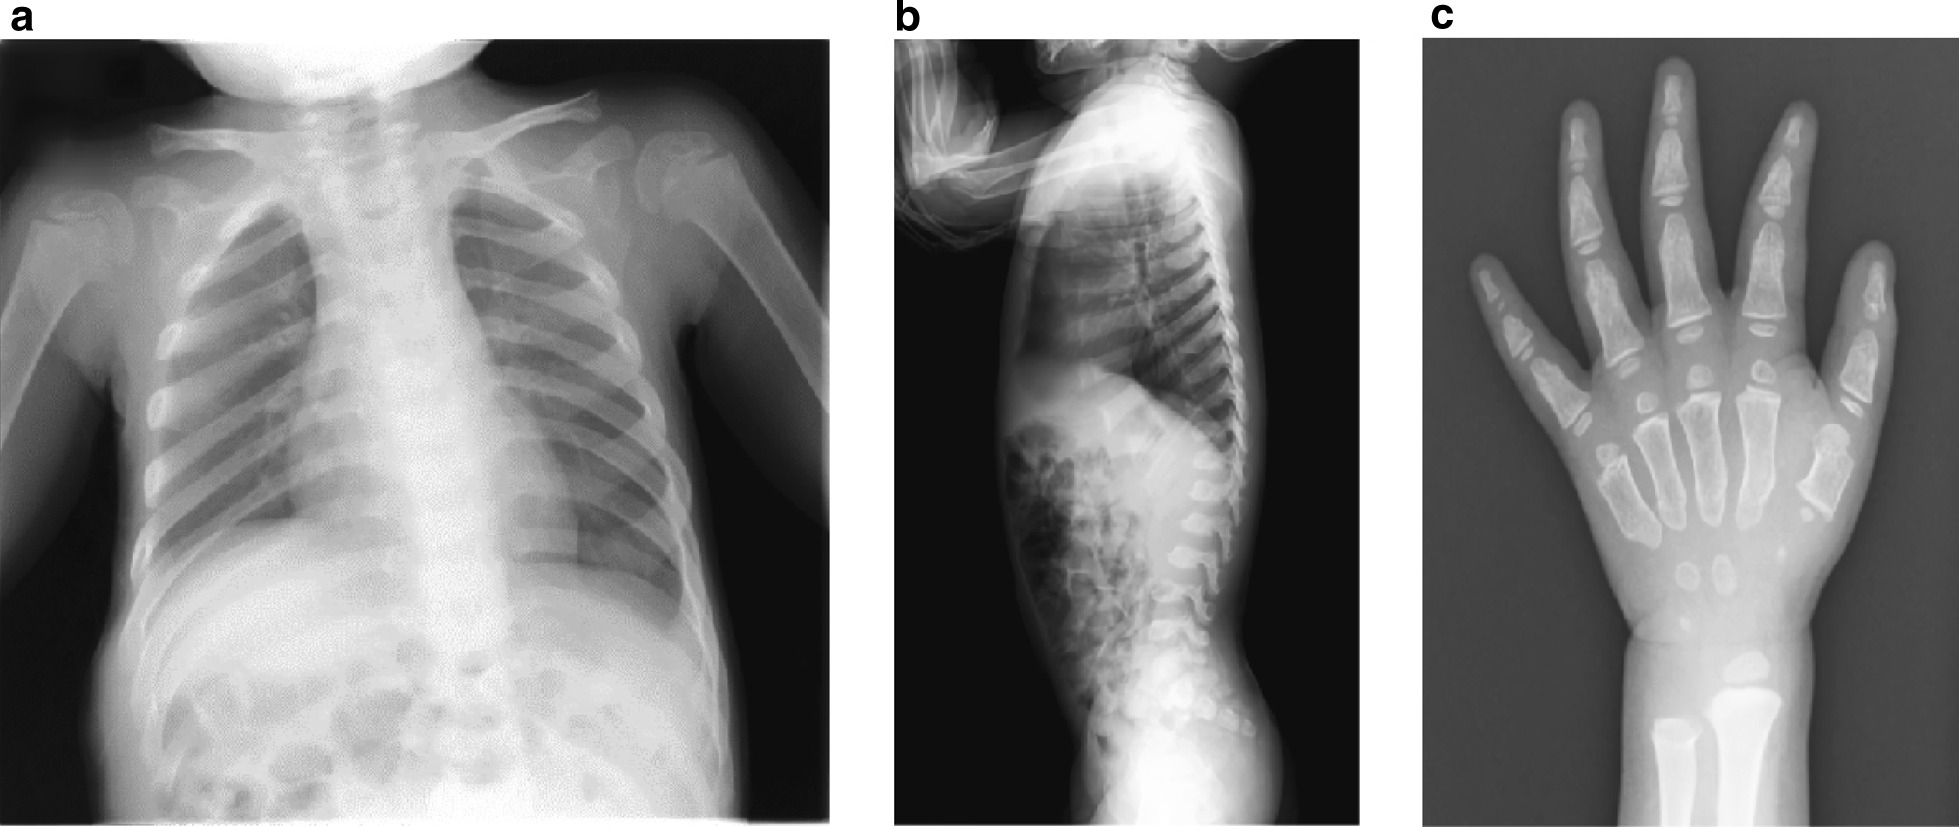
Skeletal features evident by a chest X-ray, b thoracolumbar X-ray, and c carpus X-ray. Chest X-ray showed the flattening of ribs (“oar-like” ribs). Thoracolumbar X-ray showed worsening of kyphosis and ovalization of the thoracolumbar vertebral body. Carpus X-ray showed short and stubby phalangeal bones with mild metaphyseal cupping, and strikingly short metacarpals with proximal coning.
